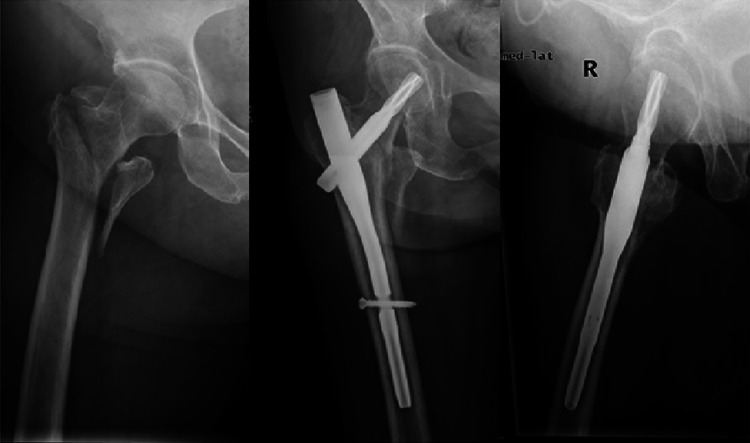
Excellent quality of fracture reduction. Slight valgus and 180° for alignment, positive cortical support and smooth anterior cortical contact for displacement in AP and lateral radiography were achieved
